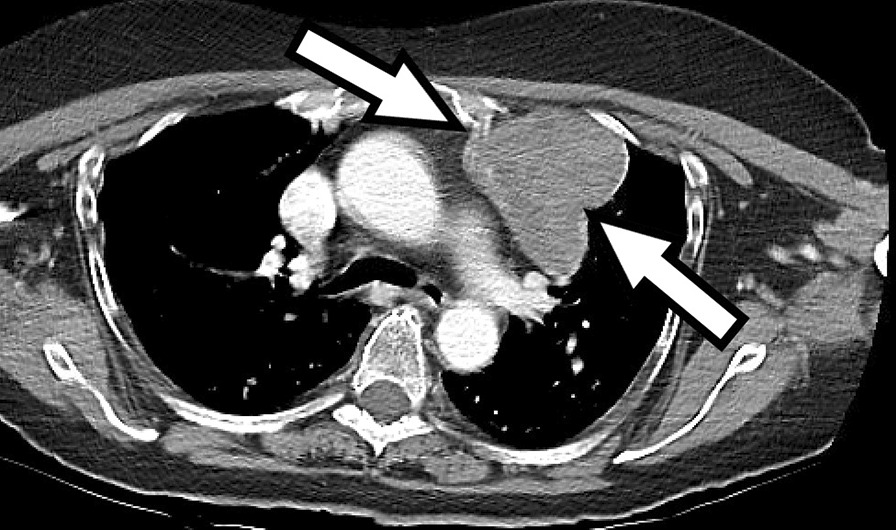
Axial CT image of the chest with IV contrast demonstrates a heterogenous, partially enhancing mass (arrow) along the anterior and mediastinal pleura
